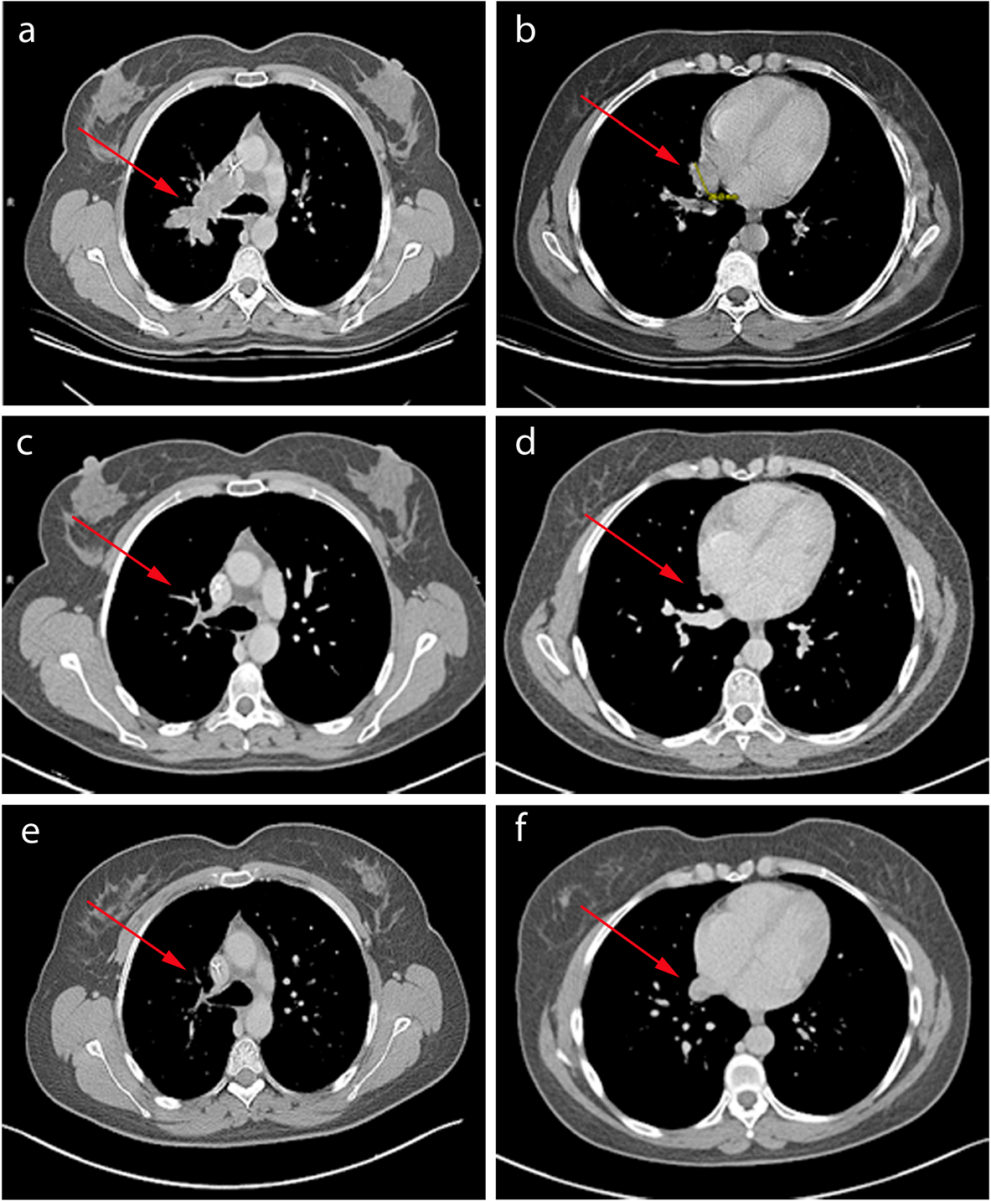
Tumor shrinkage assessed by computed tomography. (a and b) Mediastinal and chest progression without treatment in March 2009; (c and d) tumor shrinkage of 90% according to RECIST criteria in January 2011 after 10 months of Sorafenib; (e and f) stable disease in January 2013.
